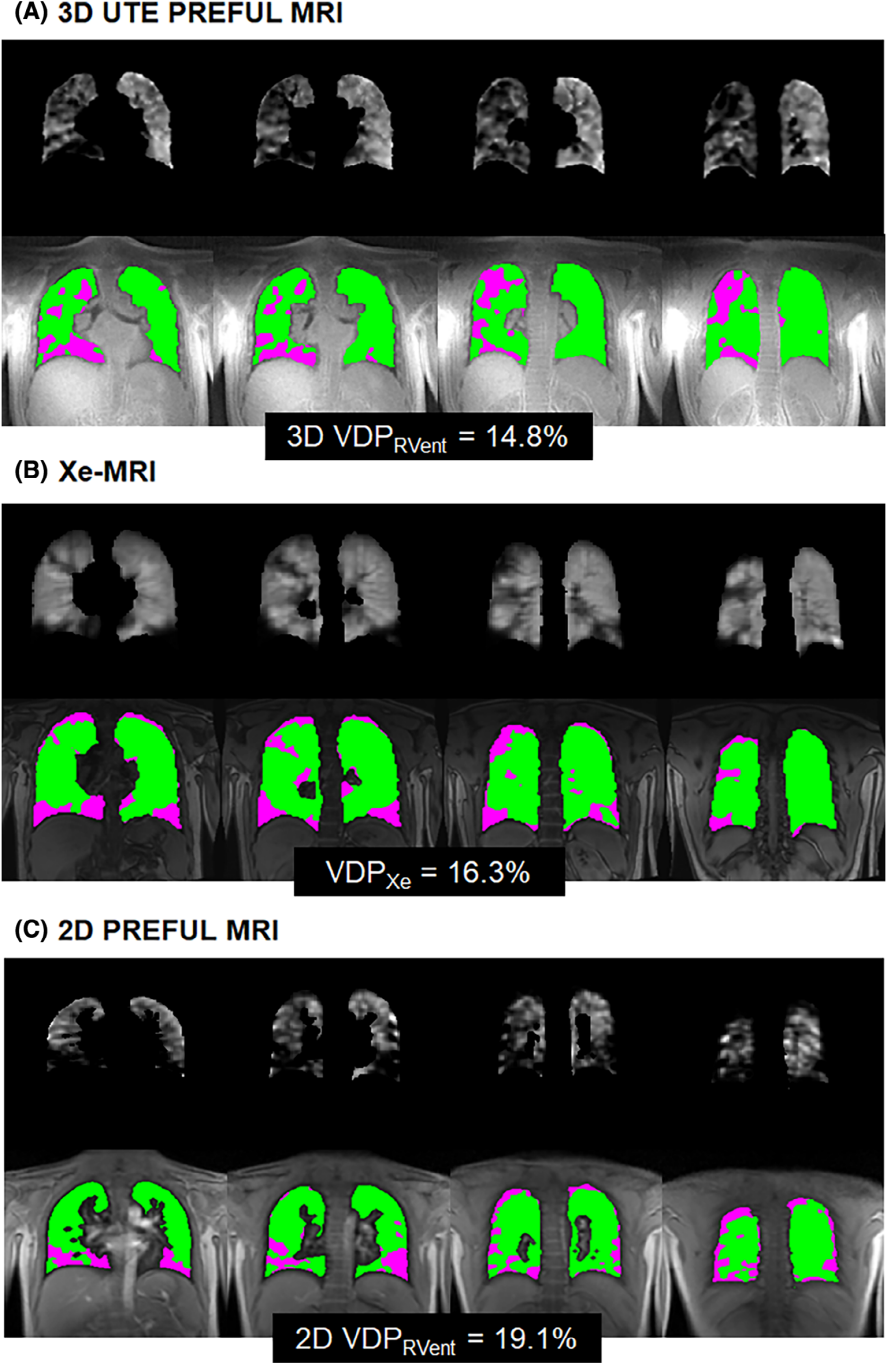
Normalized and segmented, gray‐scaled ventilation maps and corresponding defect maps derived from (A) 3D UTE phase‐resolved functional lung (PREFUL) MRI (B) ^129^Xe‐MRI (Xe‐MRI) (C) and 2D PREFUL MRI for a representative pediatric CF patient. Similar ventilation defects can be found at the inferior region of both lungs and at the superior region of the right lung toward the posterior slices. Dice coefficients for healthy and defect regions, respectively, are: 0.75 and 0.24 for 3D VDP_RVent_ and VDP_Xe_, 0.77 and 0.16 for 3D VDP_RVent_ and 2D VDP_RVent_, and 0.75 and 0.21 for 2D VDP_RVent_ and VDP_Xe_. CF, cystic fibrosis; VDP, ventilation defect percentage.
